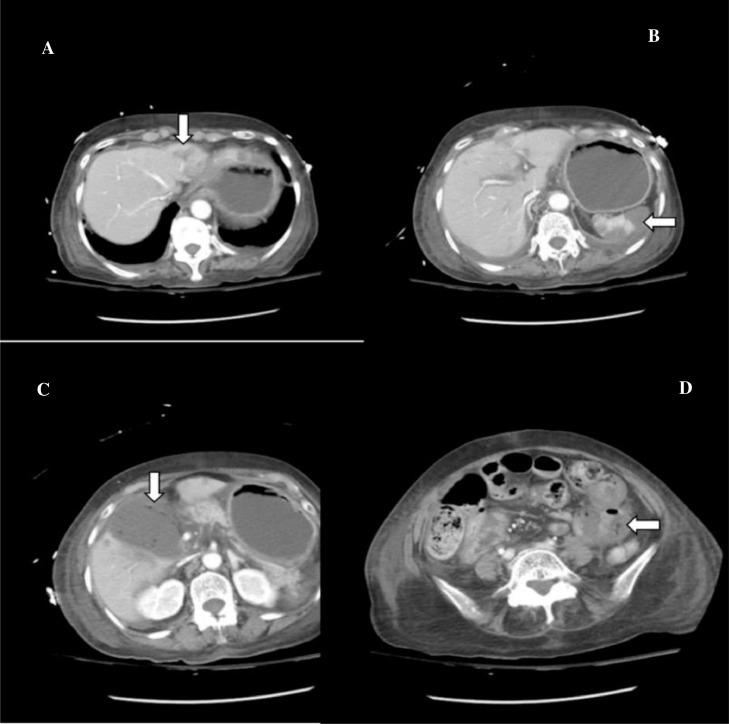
Multiple axial images from contrast-enhanced abdominal CT. (A) shows a low attenuation area in the liver representing liver infarction (white arrow). (B) shows multiple splenic infarctions (white arrow). (C) shows air specks in the wall of the gall bladder secondary to ischemia (white arrow). (D) shows the absent enhancement of small bowel loops secondary to mesenteric ischemia (white arrow).
